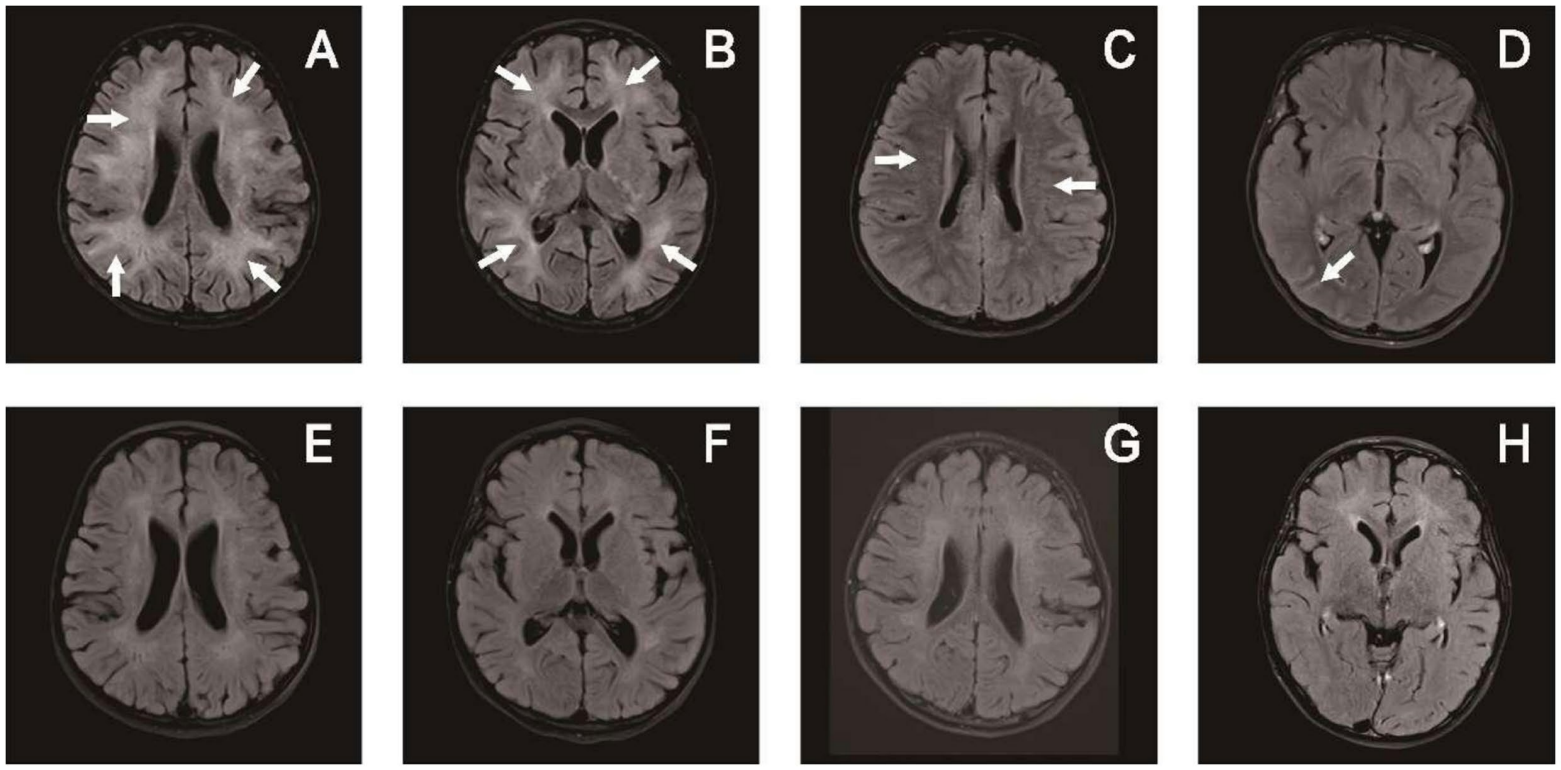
Brain MRI of patient 6 in Table 1: upon admission (A–D) and 3 months later (E–H). (A,B) T2-hyperintense lesions in the white matter of bilateral cerebral hemispheres. (C) Periventricular radial linear enhancement. (D) Enhanced shadow of occipital lobe sulci. (E–H) Follow-up images of improved T2 lesions and enhancement.
